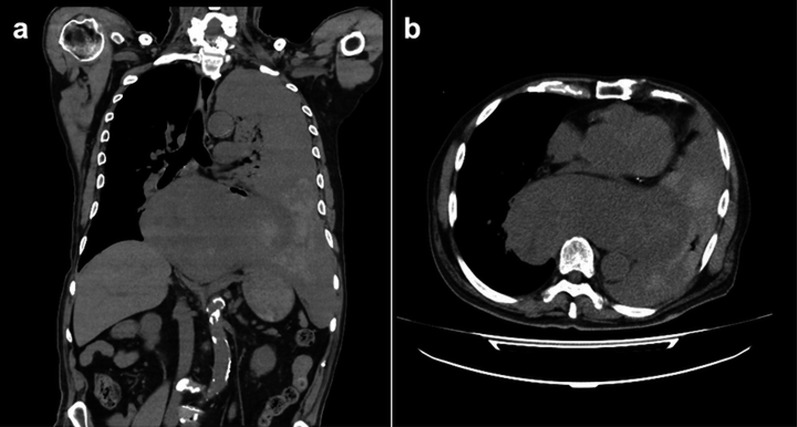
Plain computed tomography of the chest showing mediastinal enlargement and left hemothorax: a coronal, b axial
